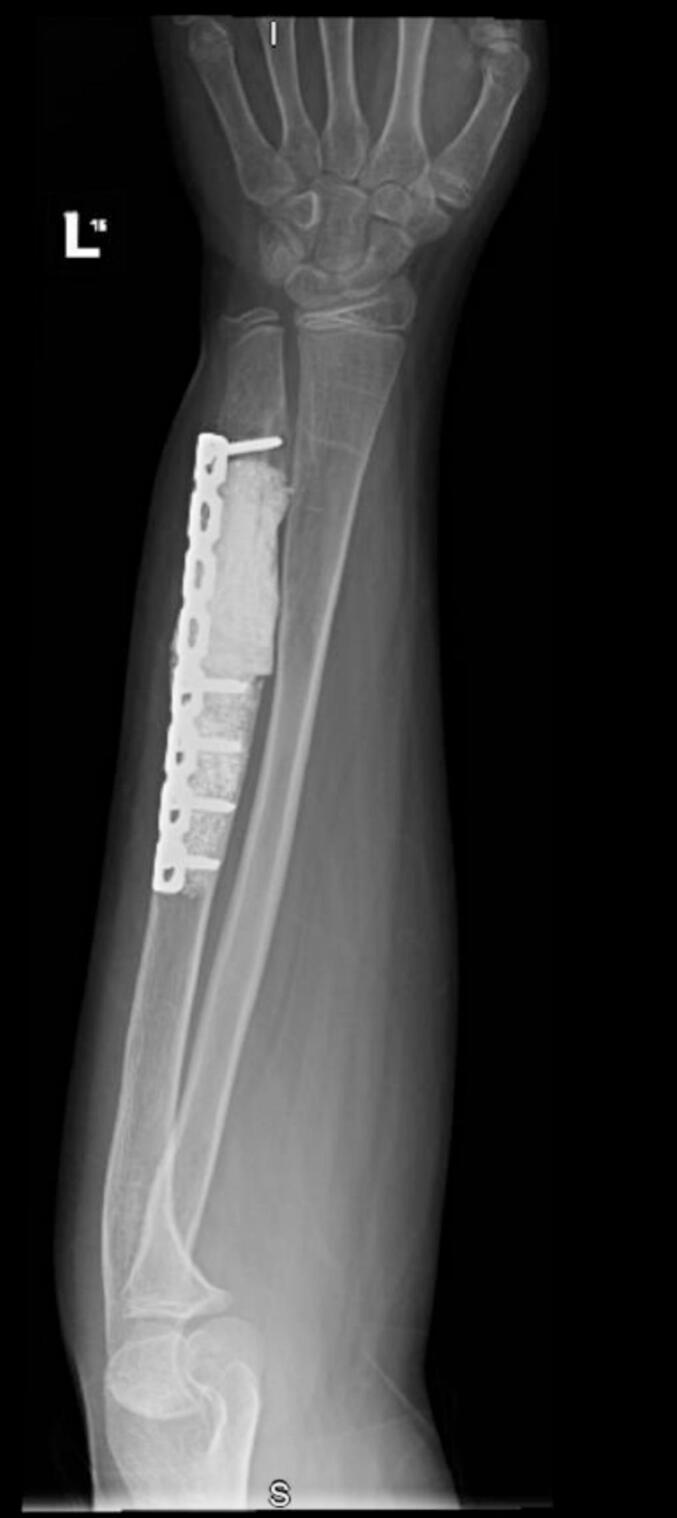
Radiograph obtained 5 years postoperatively; Anteroposterior view of the left forearm demonstrating stable cement, plate, and screw fixation at 5 years after lesion resection.
